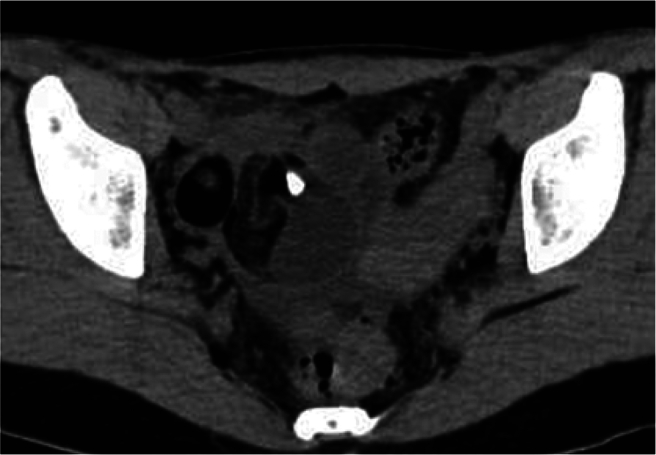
Right ovarian teratoma on CT scan. Preoperative non-contrast-enhanced CT shows a right ovarian teratoma, 5 cm long, containing fat and a calcified part, which had been 3 cm long one year previously, as seen in Fig. 1.
